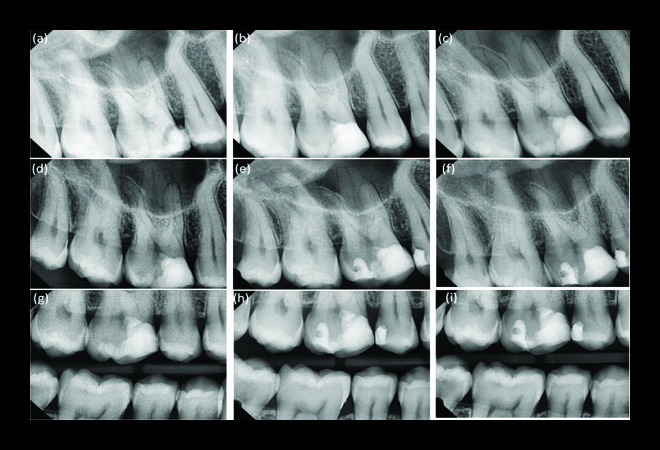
(a) Diagnostic periapical radiograph. (b) After partial pulpotomy. (c–f) 6-, 12-, and 18-month postoperative periapical radiographs, respectively, showing normal periapical tissues. (g) Two-year postoperative bitewing radiograph showing caries in the distal occlusal surface. (h, i) Three-year and four-year postoperative bitewing radiographs, respectively, showing no evidence of mineralized bridge formation.
